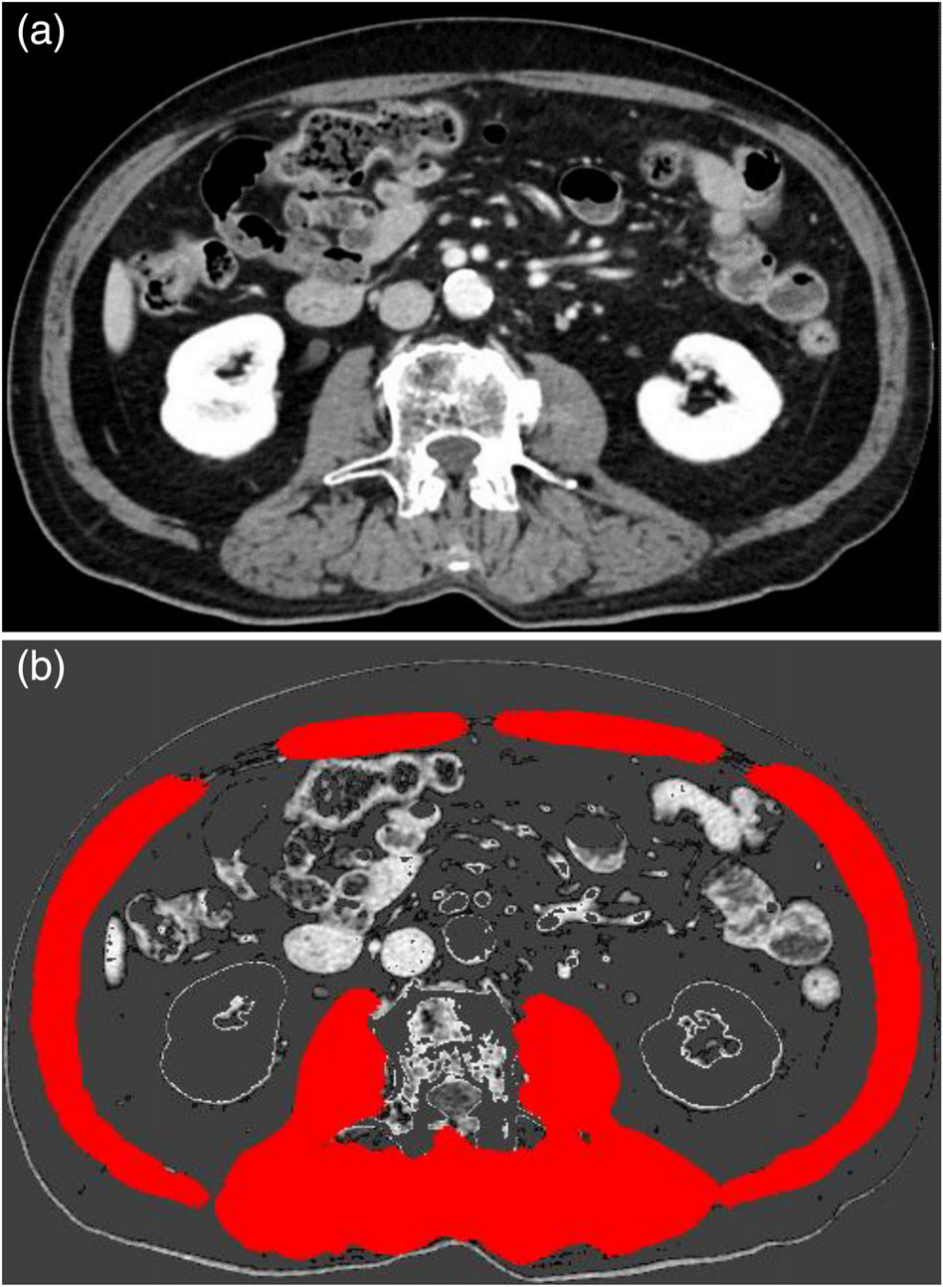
Method for measuring skeletal muscle mass at the third lumbar vertebra. (a) The computed tomography image was taken from the picture archiving and communication system and was saved in digital imaging and communications in medicine (DICOM) format. (b) The image was post‐processed using in‐house developed software for calculation of cross‐sectional area of abdominal wall and back muscles, as delineated in red, to calculate the third lumbar vertebra skeletal muscle area (SMA). The identification and quantification of skeletal muscle relied on Hounsfield unit (HU) thresholds, spanning from −29 to +150 HU.
